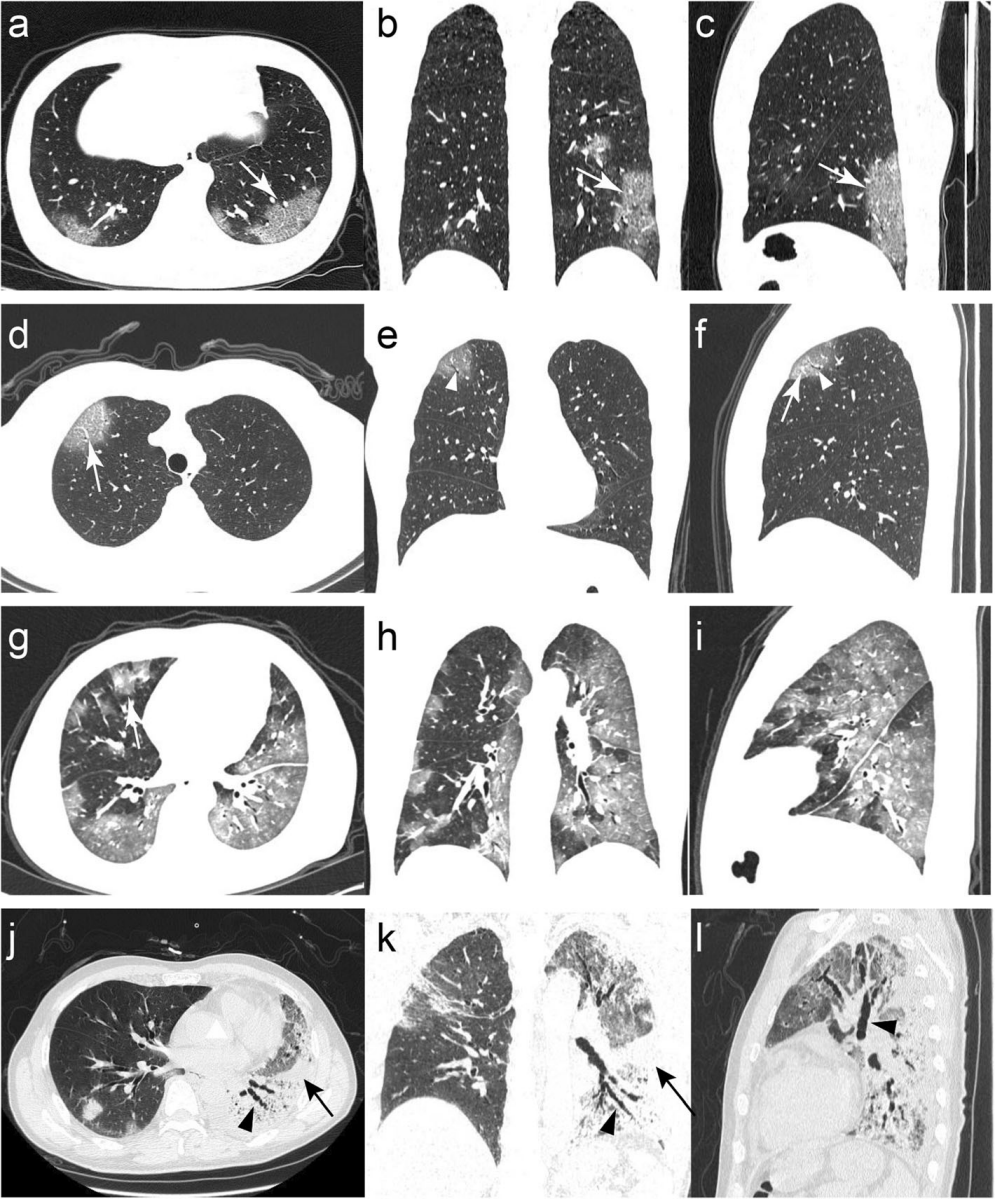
Thin-section CT images for severe and non-severe patients. a-c Images of a 25-year-old woman with non-severe COVID-19 pneumonia (CT score = 2) who had the symptoms of dry cough and fever. The axial, coronal and sagittal CT images all presented subpleural GGO (with craving stone sign) in the lower lobes of both lungs (white arrows). d-f Images of a 55-year-old woman with non-severe COVID-19 pneumonia (CT score = 1) who had the symptom of fever. The axial, coronal and sagittal CT images all presented GGO in the anterior segment of the upper lobe of the right lung, containing air bronchogram (white arrowheads) and vascular thickening (white arrow). g-i Images of a 52-year-old man with severe COVID-19 pneumonia (CT score = 4) who had the features of fever and comorbidity (diabetes, hypertension). The axial, coronal and sagittal CT images showed diffuse large regions of GGO with partial consolidation and interlobular septal thickening (white arrow). j-l Images of a 64-year-old man with severe COVID-19 pneumonia (CT score = 4) who had the symptoms of fever and cough. The axial, coronal and sagittal CT images showed diffuse large regions of GGO, accompanying consolidation (black arrows), and beaded air bronchogram (black arrowheads)
